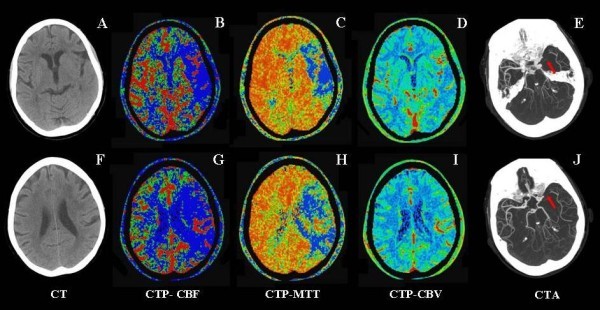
CT protocol performed 3 h after stroke onset. *A and F*: unenhanced CT scans (ASPECTS levels) show the presence of an area of slight hypoattenuation in the left insular ribbon and in the ipsilateral frontal cortex (ASPECTS = 8); *B-D and F-H*: CTP maps (ASPECTS levels) show the occurrence of a large mismatch CBF > CBV or MTT > CBV in the territory of left middle cerebral artery; *E and J*: axial CTA MIP images document the occlusion of M1 segment of left middle cerebral artery (*arrows*).
